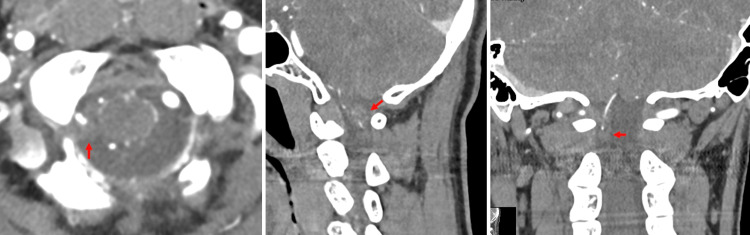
CT angiography showed no apparent abnormal vessels around the hematoma: (A) axial, (B) sagittal, and (C) coronal views. Red arrows indicate hematoma.
